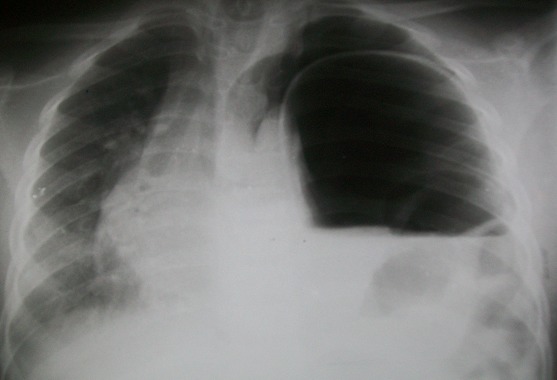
Radiographie thoracique préopératoire
